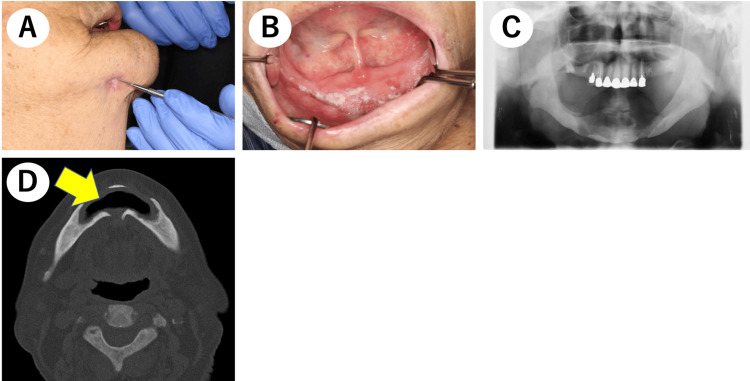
Clinical and radiographic findings after the remaining sequestrum was completely shed naturally. (A) The extraoral examination revealed a fistula but no signs of pus drainage.
